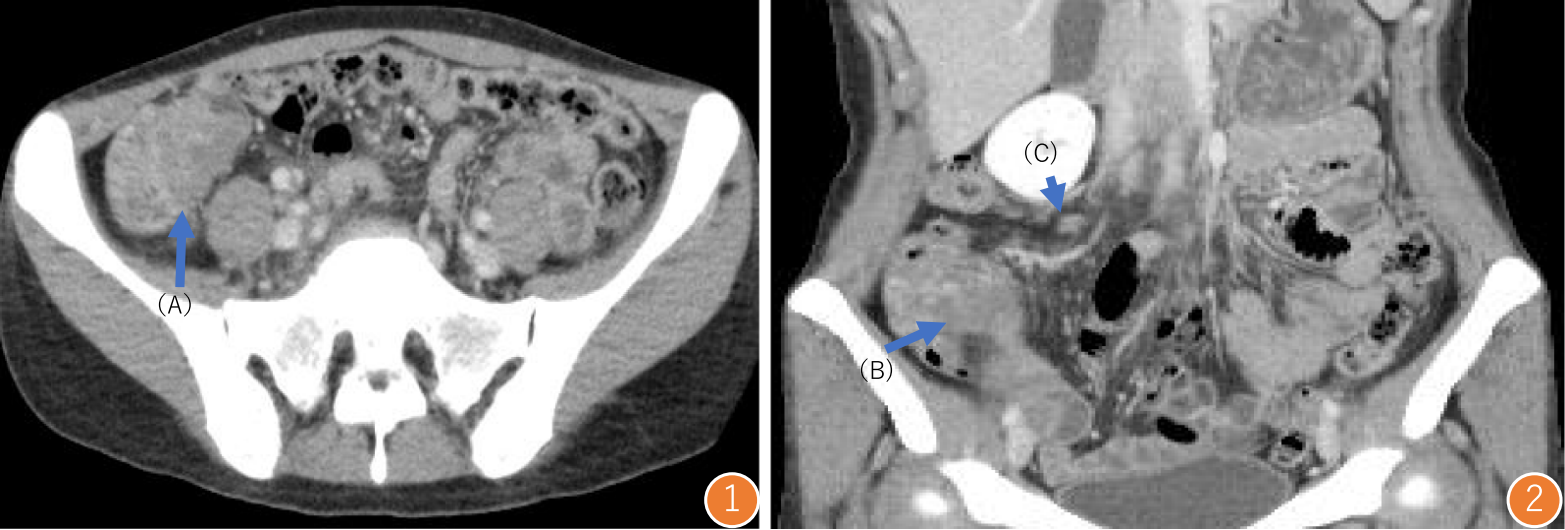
Contrast-enhanced CT. ①: Axial view: A thickness of the cecal wall. ②: Coronal view: B thickening of the cecal wall is observed. C Enlarged regional lymph nodes are seen
